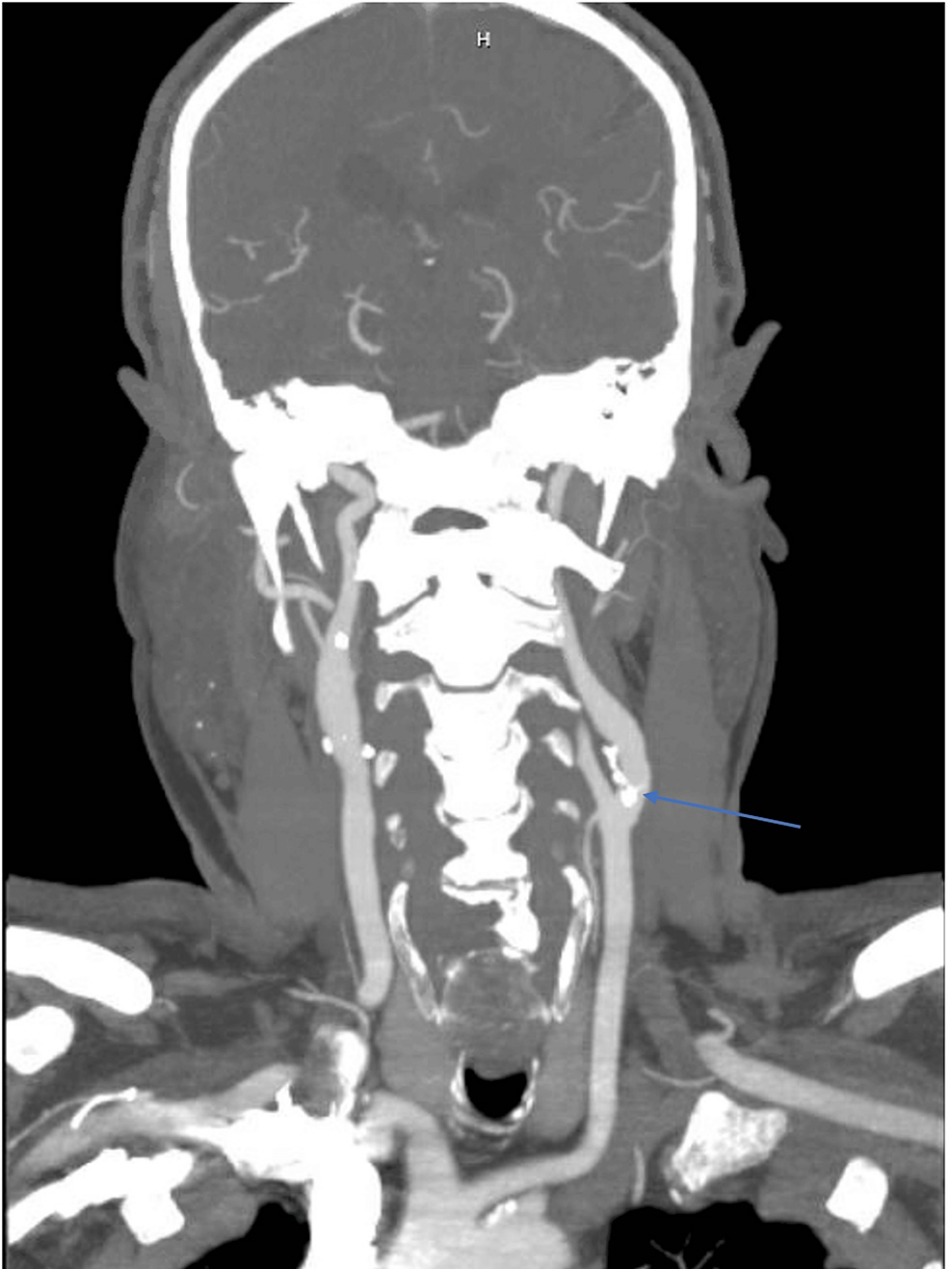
CTA of the head and neck vessels (coronal maximal intensity projection) demonstrating mixed calcified and soft plaque in the proximal left internal carotid artery protruding from the posterior wall and extending more centrally with no gross hemodynamically significant stenoses (arrow). CTA: CT angiography
